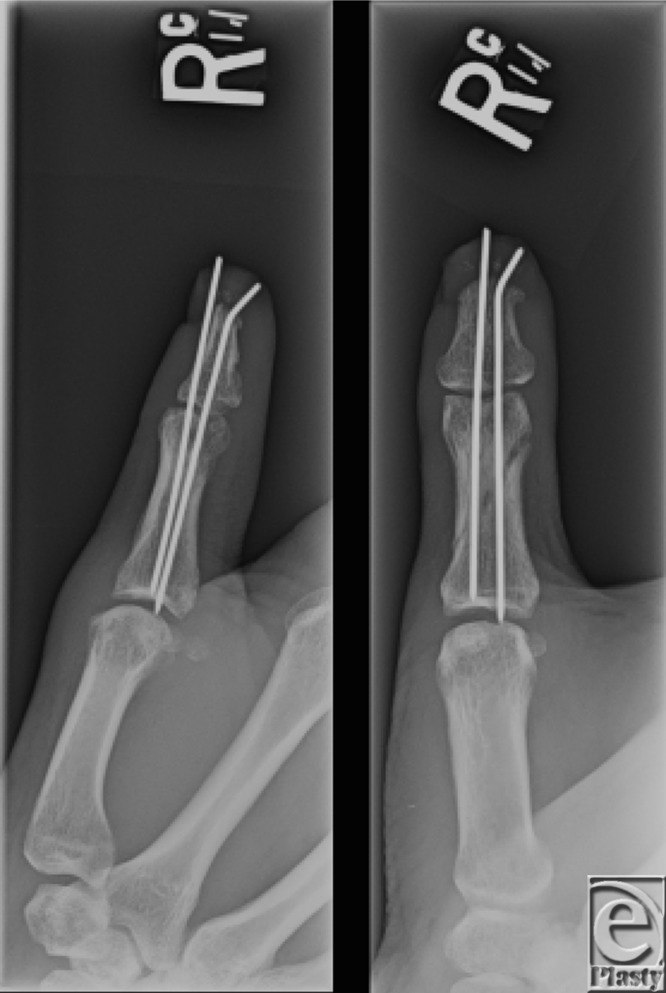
Intraoperative X-ray showing approximately 2- to 3-mm loss of distal phalanx, and adequate reduction of the proximal phalanx fracture after pinning.
